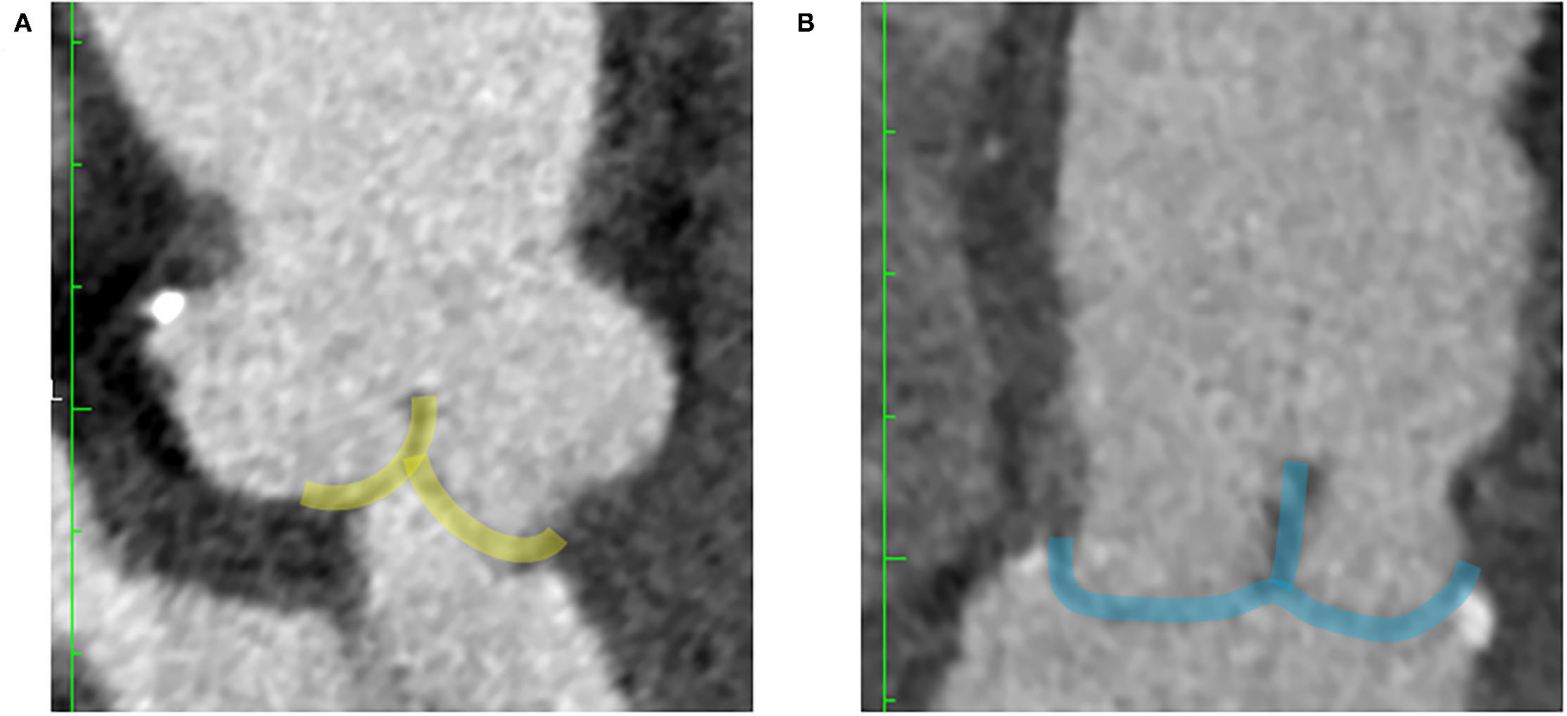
CT features of post-repair valve coaptations. The shaded green area delineated the post-repair coaptation without leaflet billowing in a reversed Y form (A). The shaded blue area delineated the post-repair coaptation with leaflet billowing in a reversed T form (B).
